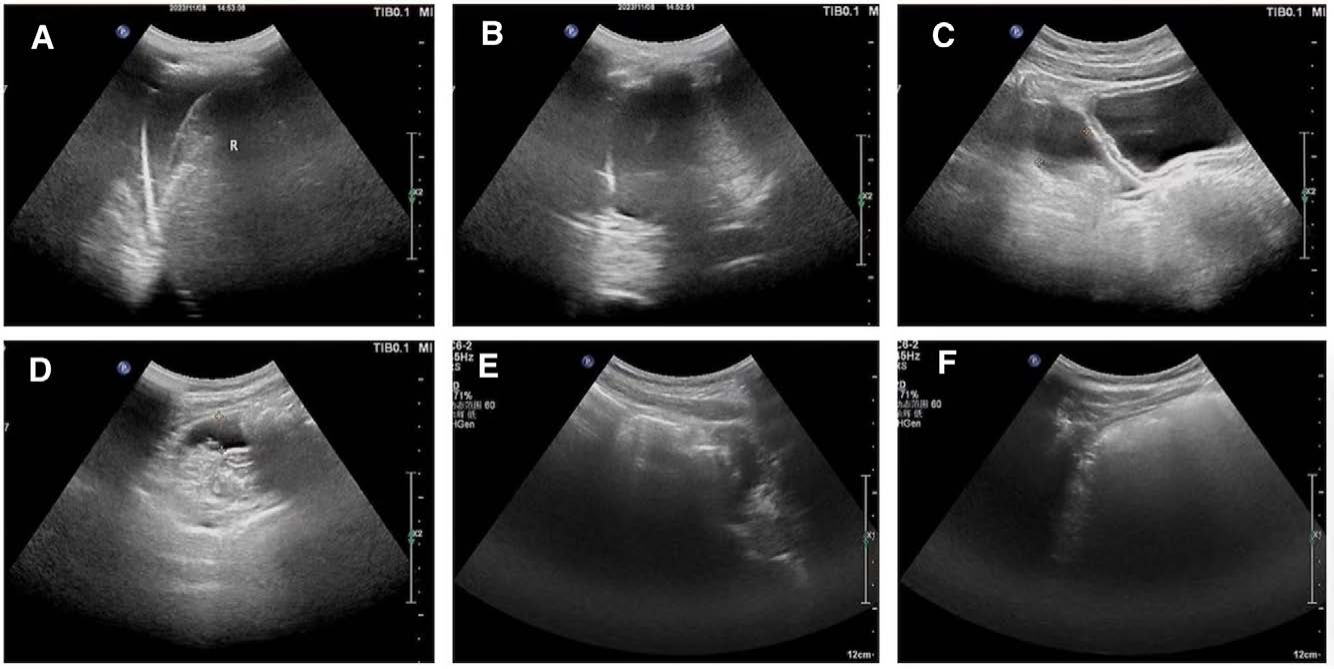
(A–D) Ultrasound during the acute phase of disease revealed bilateral pleural effusion, ascites, and pelvic effusion. (E, F) Ultrasound showed a gradual resolution of the peritoneal effusion in response to therapy.
